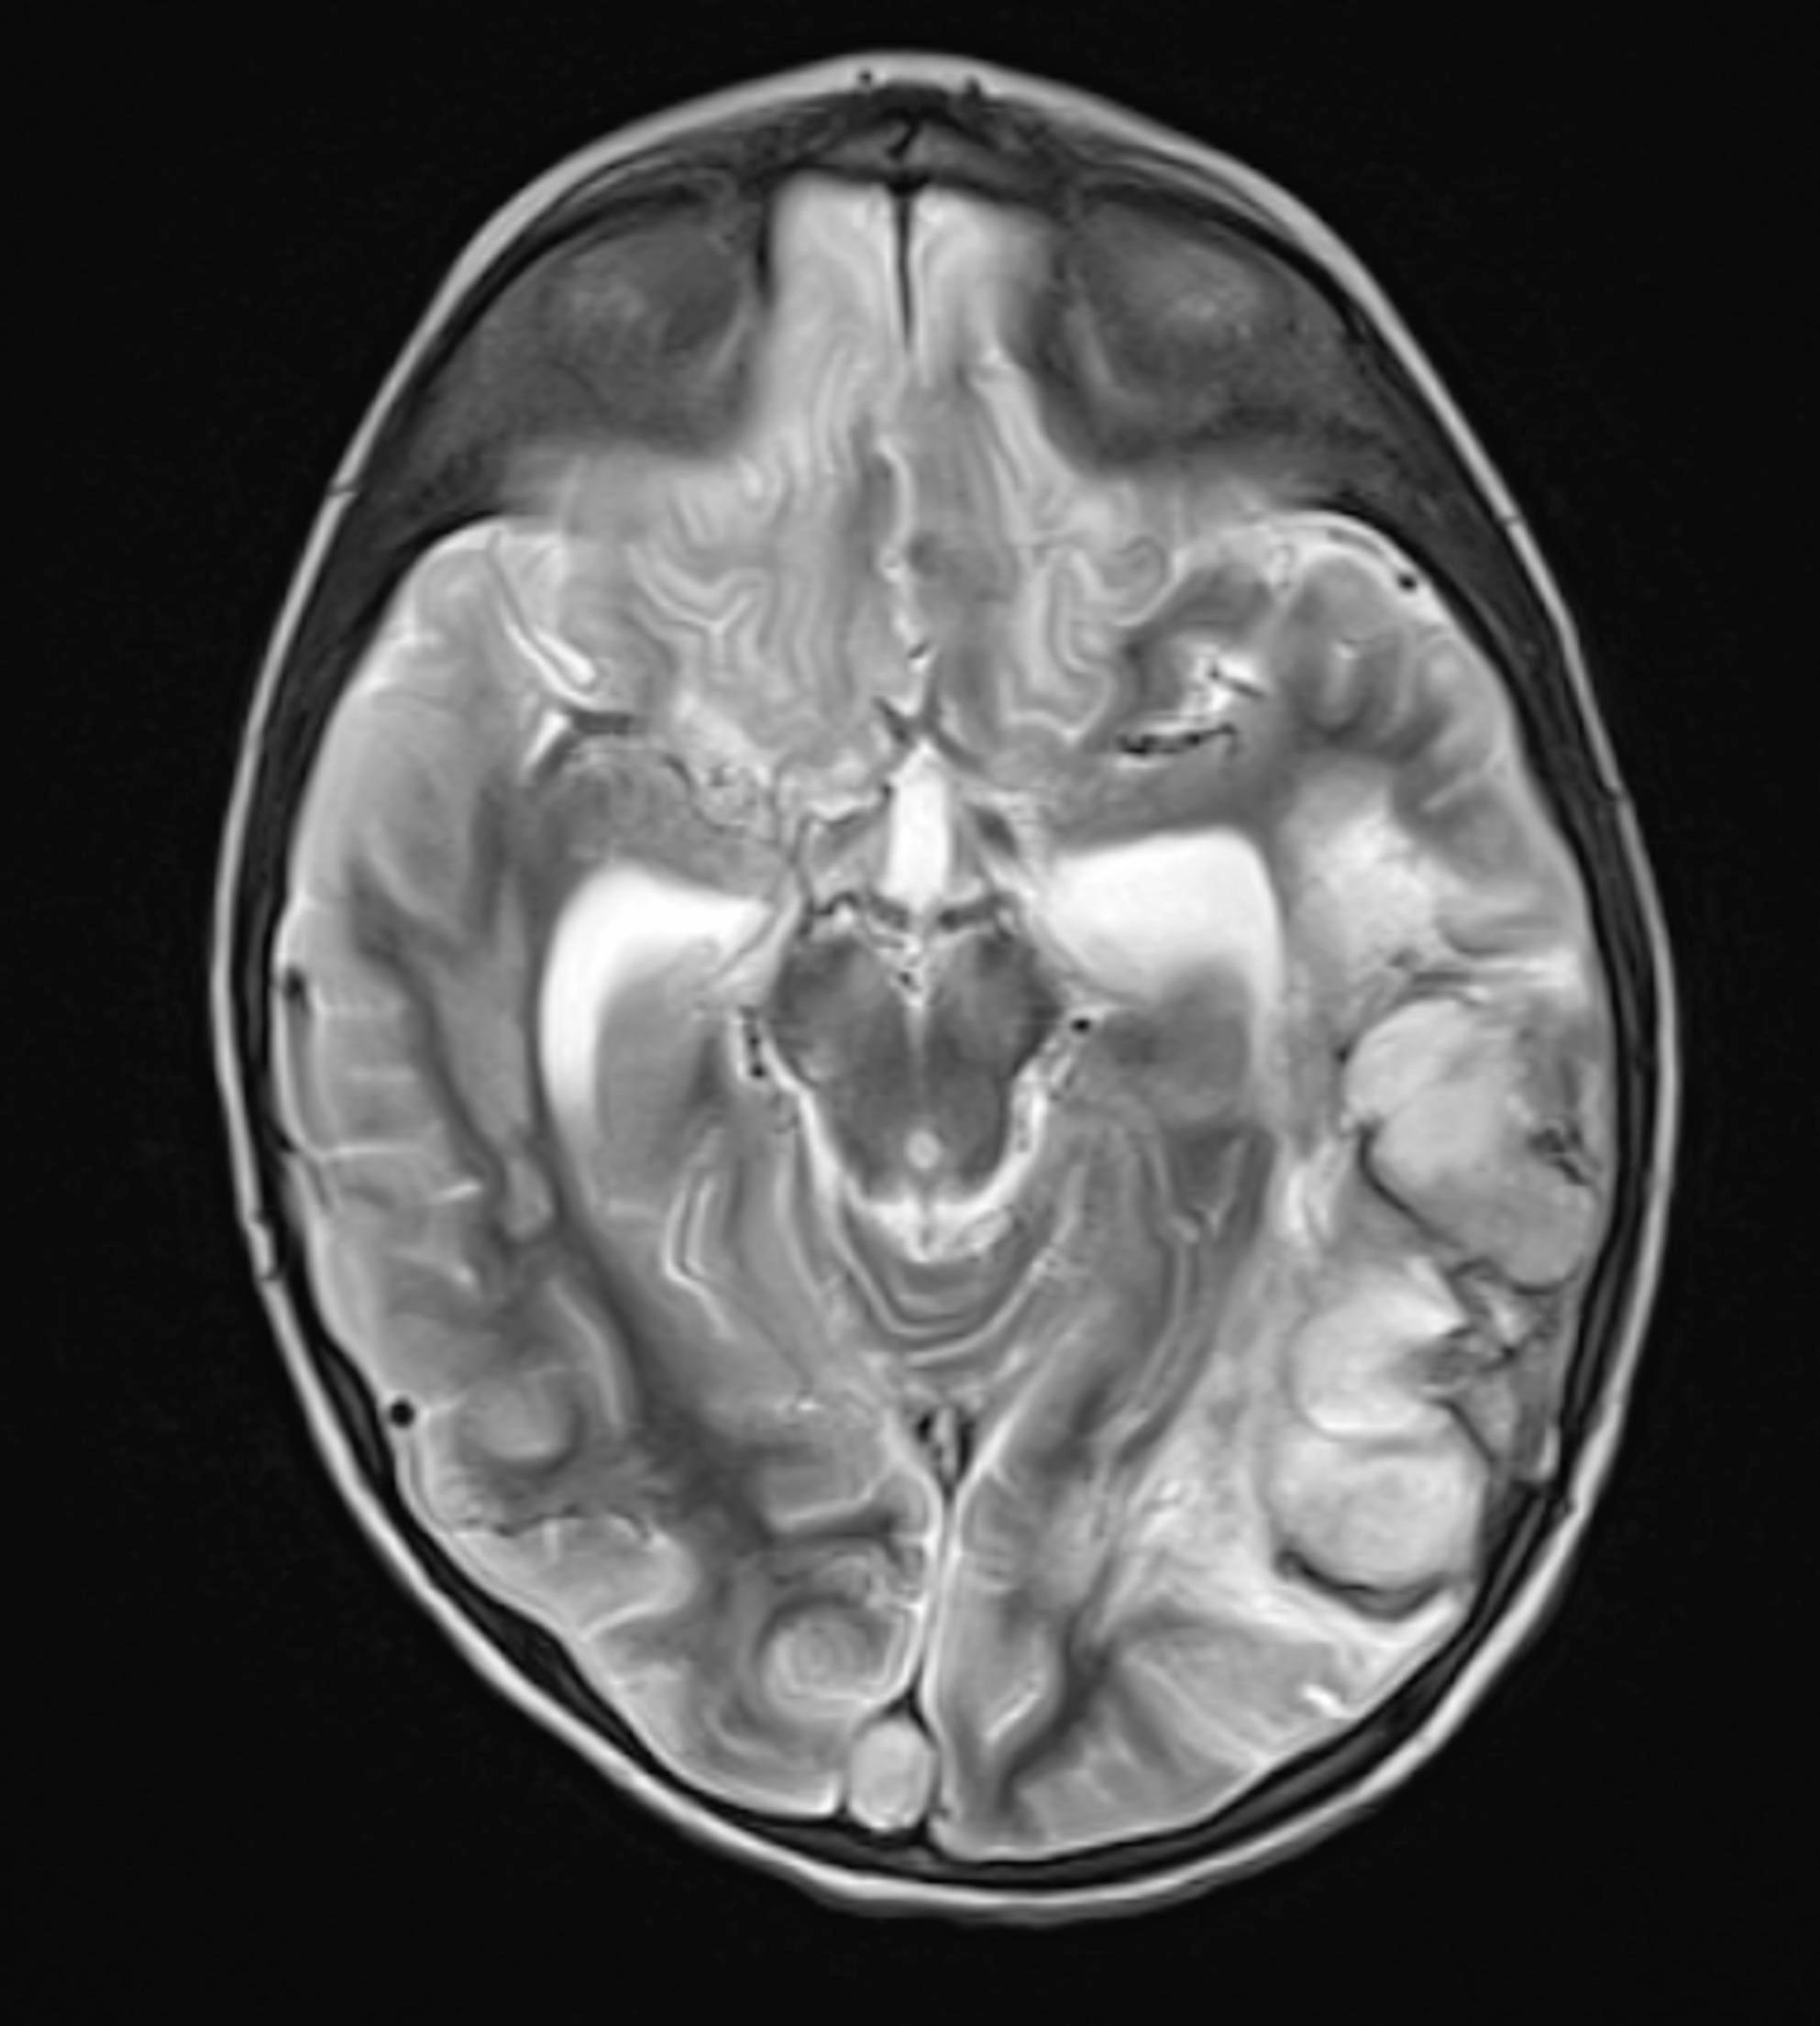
Axial T2-weighted image demonstrates diffuse hyperintense infiltrations involving the cerebral cortex, deep gray matter, white matter, and mesencephalon
